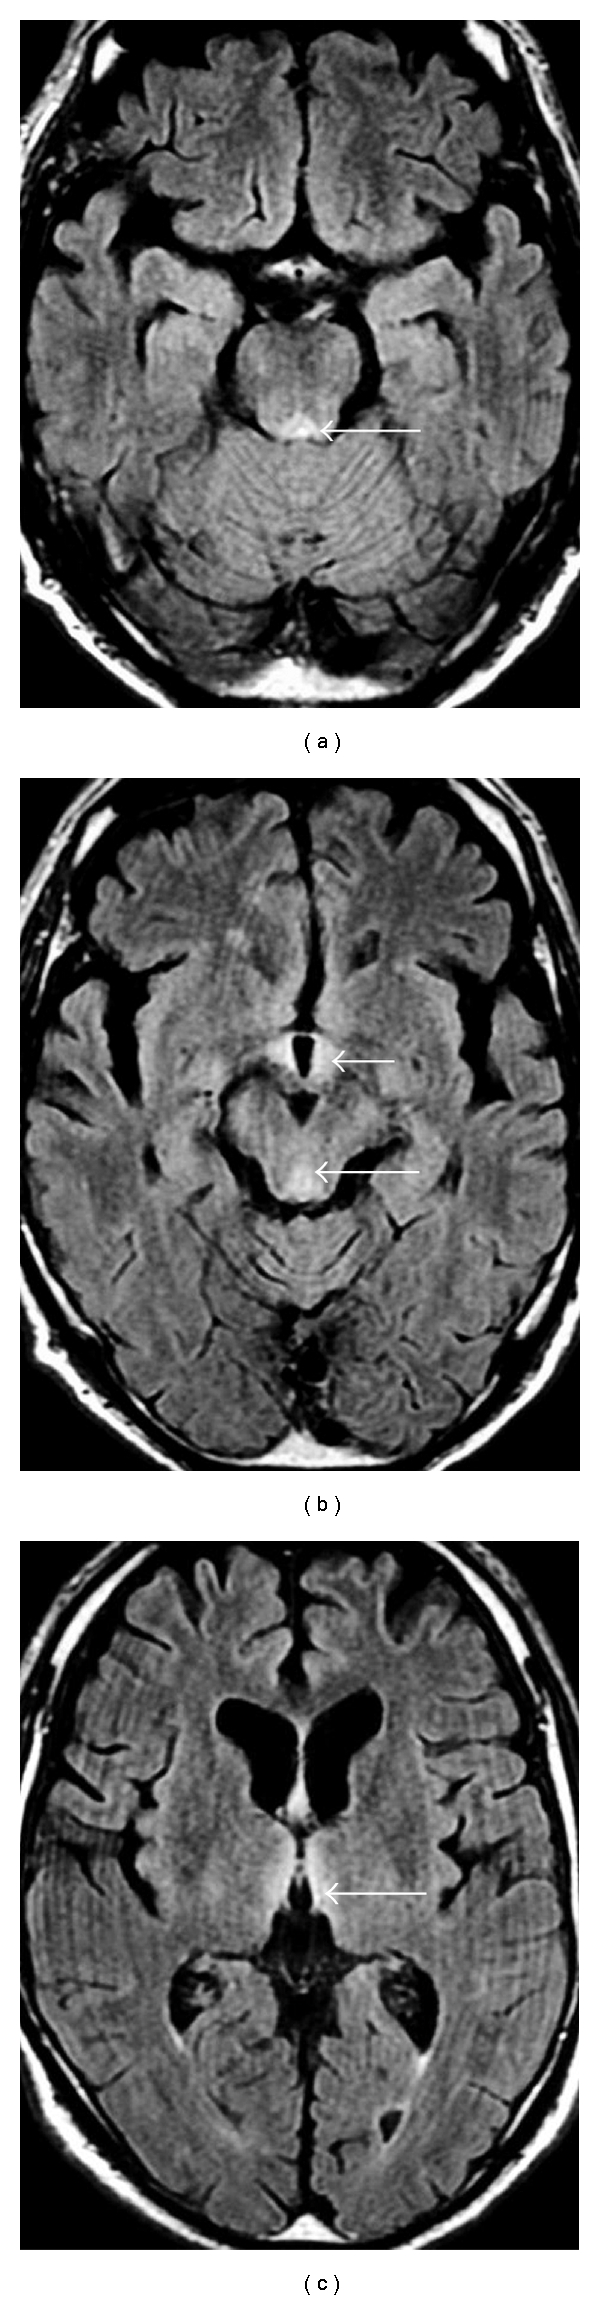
MRI-FLAIR images of the brain showed symmetrical high-intensity lesions (arrows) in the floor of the fourth ventricle (a), periaqueductal grey matter (b), hypothalami (b), and medial thalami (c).
